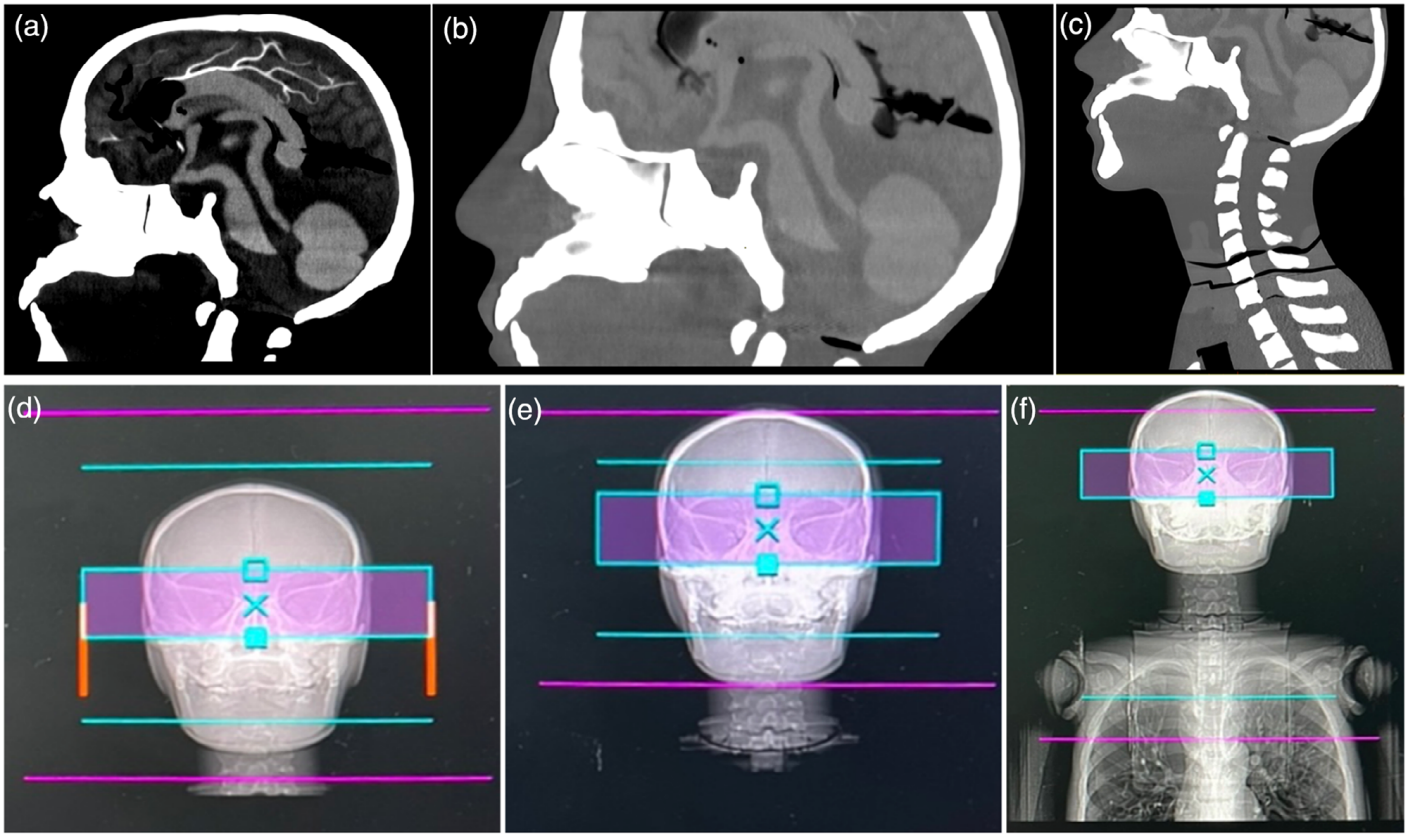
CT scan ranges and ODM activation zones for head, PNS, and C‐spine examinations. (a) Head CT scan ranges from the vertex to the skull base. (b) PNS CT scan ranges from above the frontal sinuses to the hard palate. (c) C‐spine CT scan ranges from the skull base to the first thoracic vertebra. (d–f) Activated ODM zones (pink bands) are positioned to cover the orbital level in head, PNS, and C‐spine CT examinations, respectively.
